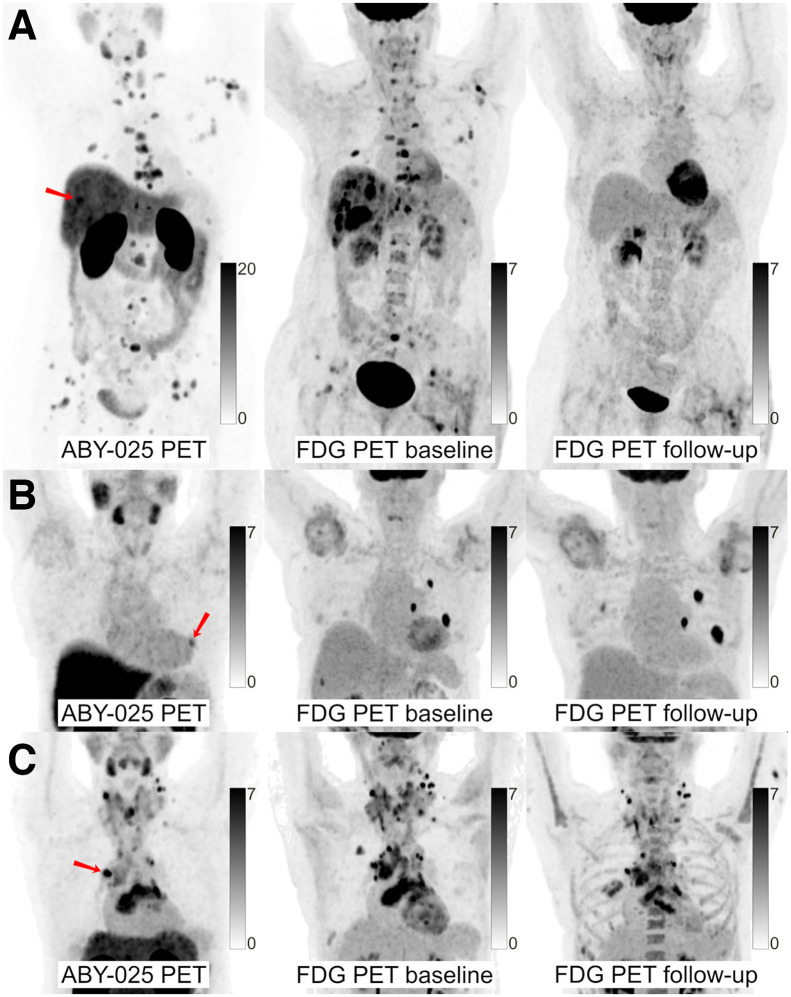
[^68^Ga]Ga-ABY-025 PET/CT and [^18^F]F-FDG PET/CT images at baseline with [^18^F]F-FDG PET/CT follow-up after 2 cycles of treatment in biopsy-confirmed HER2-positive disease. (A) Patient with high [^68^Ga]Ga-ABY-025 uptake (SUV_max_, 21), who previously received 3 lines of treatment. [^18^F]F-FDG PET/CT follow-up showed complete metabolic response. (B) Patient with low uptake (SUV_max_, 5.4), who previously received 3 lines of treatment. [^18^F]F-FDG PET/CT follow-up showed disease progression (Δ-TLG, +68%) despite HER2-targeted treatment. (C) Patient with heterogeneous [^68^Ga]Ga-ABY-025 uptake, who previously received 7 lines of treatment. [^18^F]F-FDG PET/CT follow-up showed heterogeneous response, with lesions higher in [^68^Ga]Ga-ABY-025 uptake tending to have better response. Arrows indicate biopsy sites.
